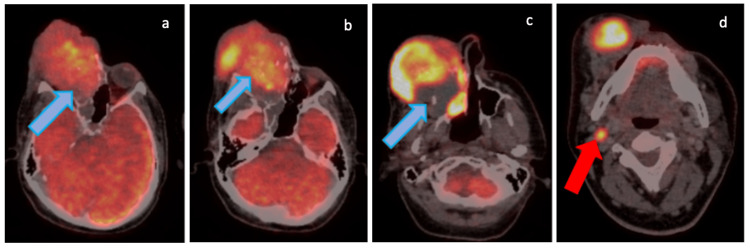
Follow-up F-18 fluorodeoxyglucose (FDG) Positron Emission Tomography (PET)/Computed Tomography (CT) (a-d) demonstrating interval increase in size and metabolic activity of the right nasal mass (solid blue arrows) and appearance of new metastatic right level II cervical lymph node (solid red arrows) suggestive of progressive disease (PD).
